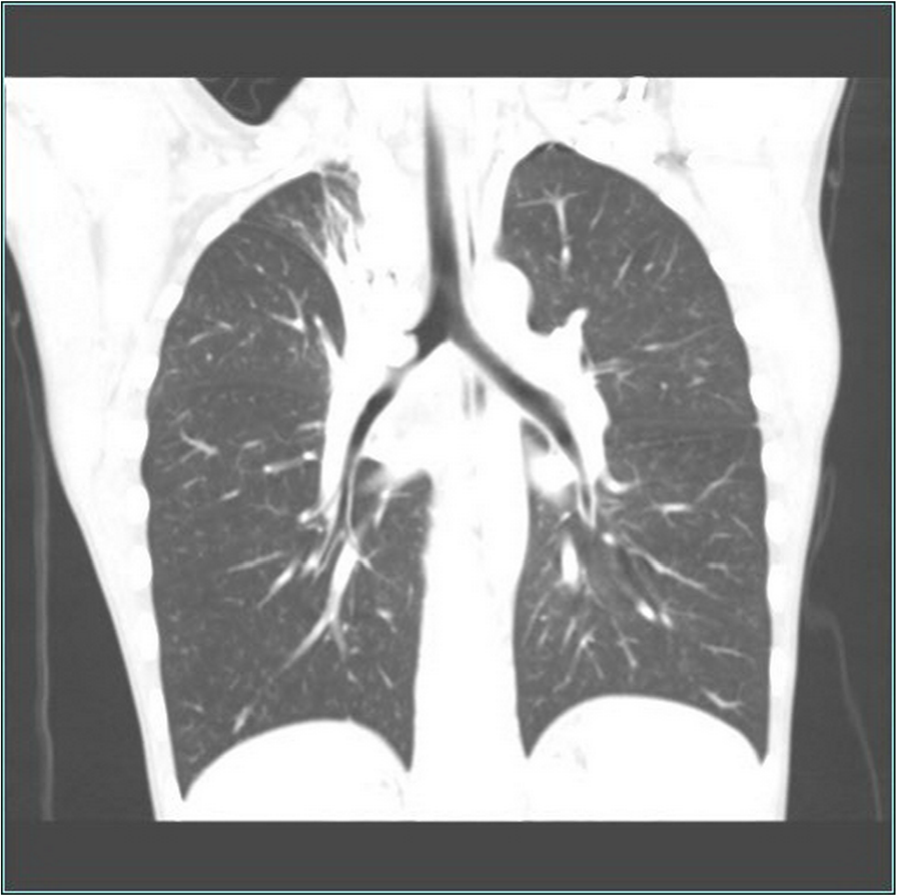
Chest CT shows a space-occupying lesion in the right principal bronchus
